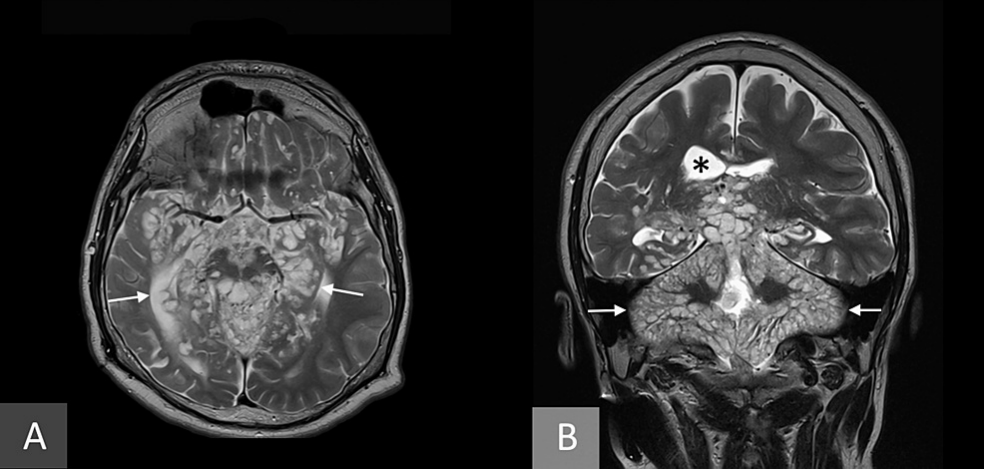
Axial (Panel A) and coronal (Panel B) T2-weighted MRI, showing extensive subpial cysts (arrows) alongside the hydrocephalus (star)
